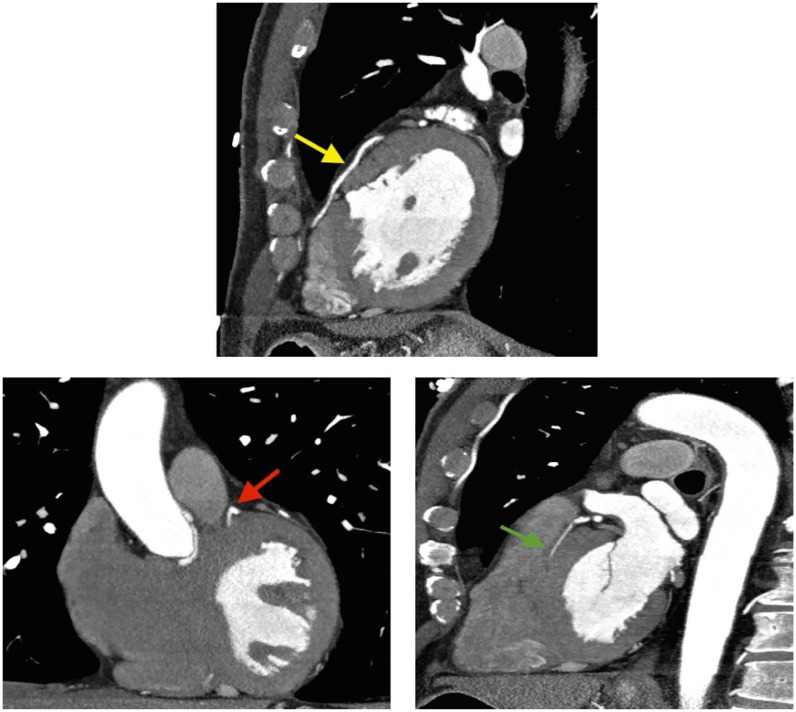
Coronary CTA with multiplanar reformatted images of the left anterior descending artery (LAD) and the diagonal branch. The LAD is a large vessel which is free of significant stenosis (Yellow arrow). There is 1 diagonal branch which has small caliber (<1 mm). The origin of this diagonal branch is patent with no plaques (red arrow). This vessel terminates abruptly raising the possibility of a small mid to distal occluded segment (green arrow).
